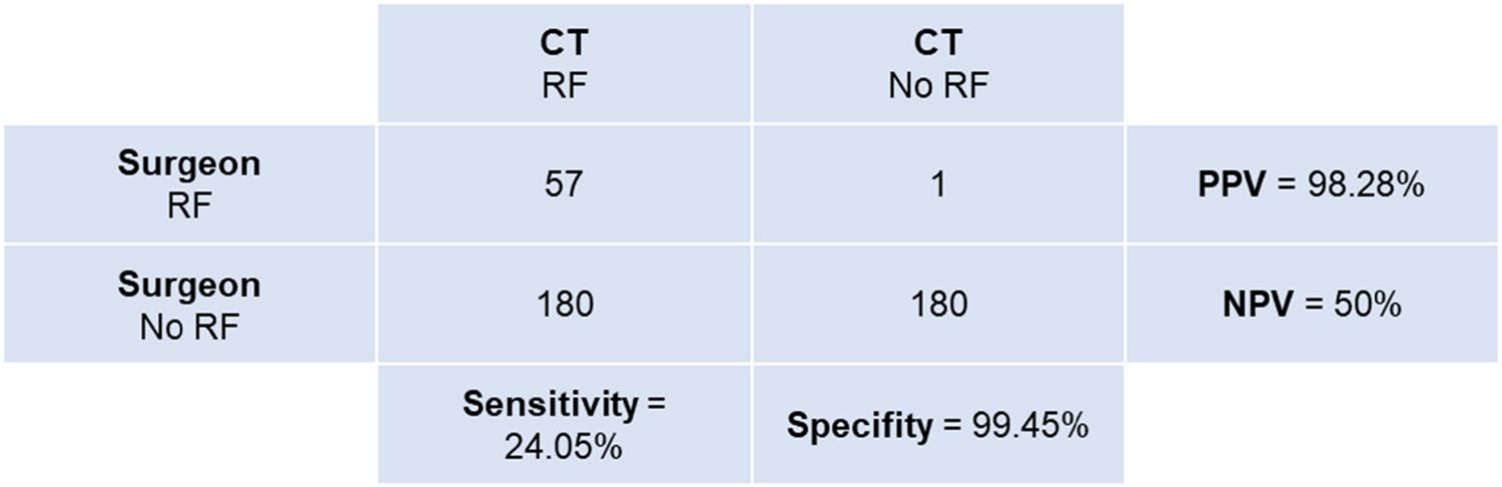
McNemar Test comparing intraoperative and CT graphic assessment of SFS
